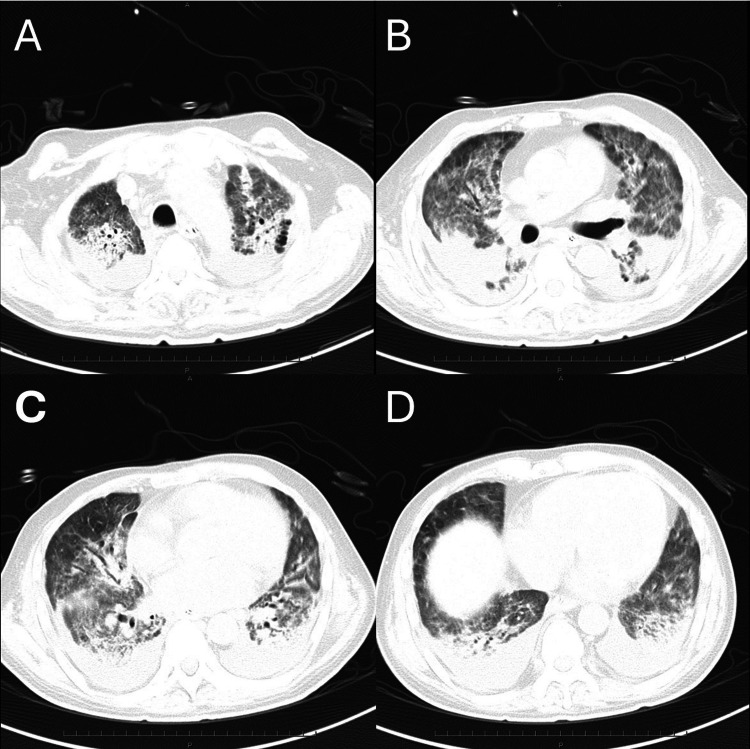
Preoperative chest computed tomography (CT) scans. Preoperative chest CT images from the apex (A) to the lung base (D) demonstrate extensive lung infiltration following severe COVID-19 pneumonia.
